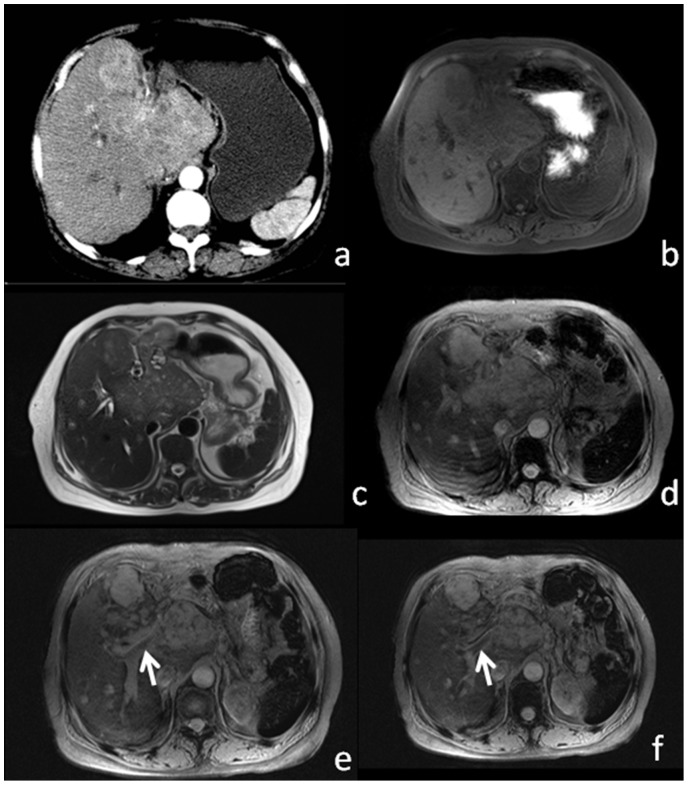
57-year-old male patient with large HCC. Enhanced CT (a) image shows patchy heterogeneous enhancement. T1WI (b) and T2WI (c) show substantial heterogeneous signal. T2*WI (d) and SWI (e) show a filling defect within the portal vein (white arrow), which corresponds to venous thrombus.
